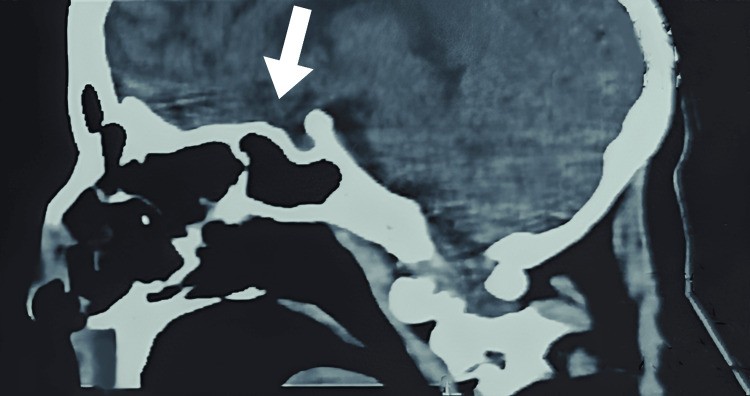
Computed tomography of the skull and orbits shows extensive sinus involvement, as pointed by the arrow.
